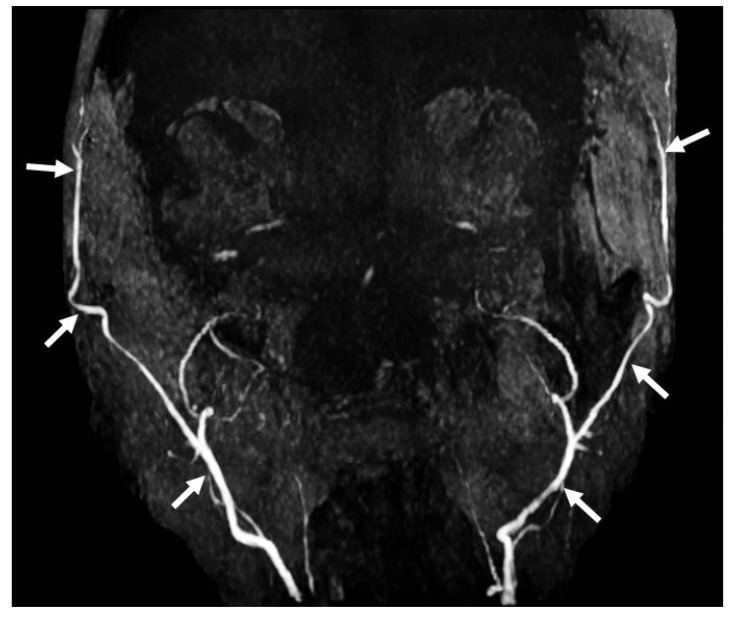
Seventy-six-year-old female with BD/DNC, 3D time-of-flight (TOF) MRI brain angiogram image shows an absence of flow in bilateral intracranial internal carotid arteries with normal flow signals in the bilateral external carotid artery and its branches (white arrows).
